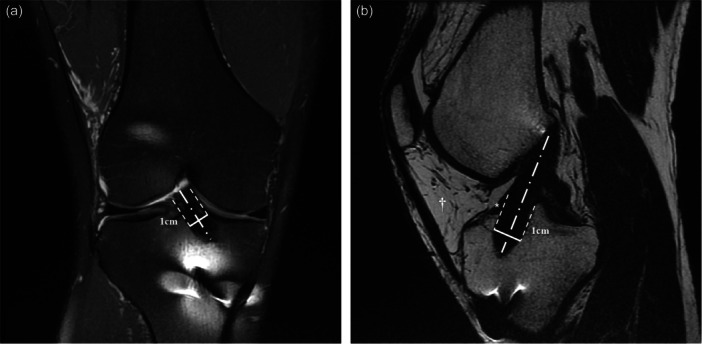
Tunnel width measurements in knee MRI. (a) Coronal view and (b) Sagittal view of the knee, showing tunnel width measured perpendicular to the tunnel's long axis, 1 cm distal to the articular surface. The tunnel's long axis is indicated by the dashed line (alternating long and short dashes), with the perpendicular tunnel width marked by the solid line. In (b), * indicates ACL remnant tissue, and † denotes the preserved fat pad. ACL, anterior cruciate ligament; MRI, magnetic resonance imaging.
